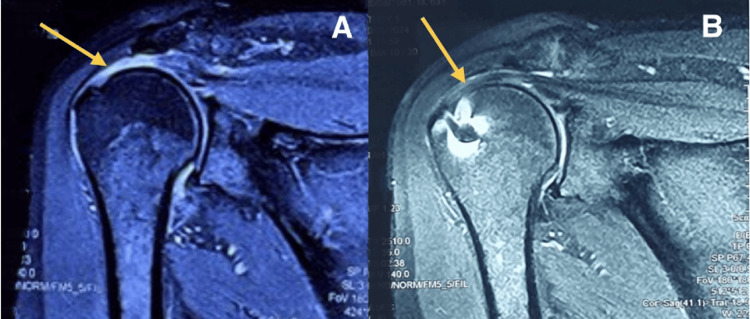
(A) Preoperative MRI of a patient with coronal section showing T2W/STIR hyperintensity (arrow) with avulsed and retracted supraspinatus tendon from the footprint. (B) MRI of the same patient done at six months postoperatively, showing tendon healing with sufficient thickness (Sugaya type I), as shown with the arrow. T2W/STIR: T2-weighted short-tau inversion recovery imaging.
